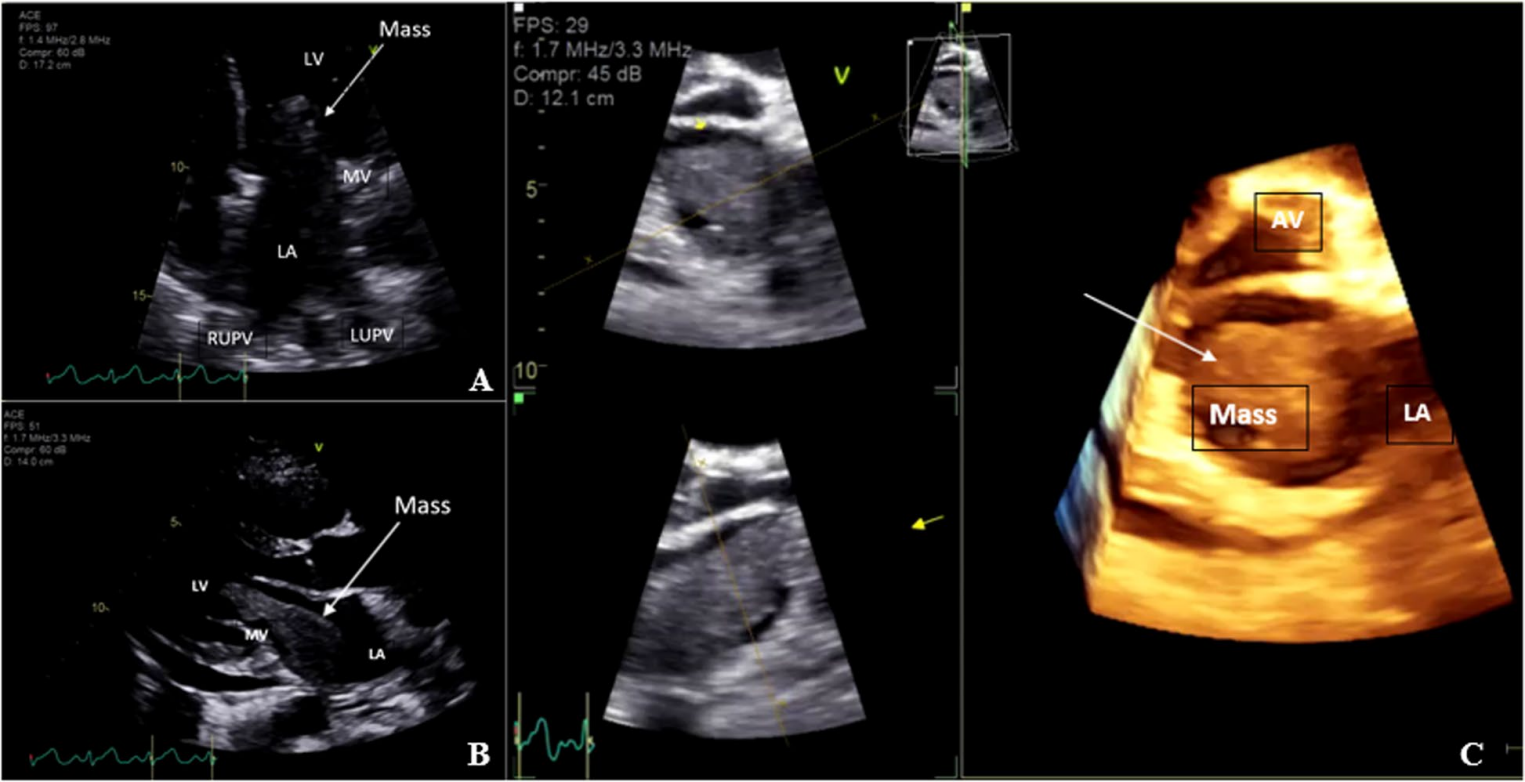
Transthoracic echocardiographic images of the left atrial mass. A 2D-echocardiography: Four chamber view demonstrates a large, echogenic mass attached to the lateral left atrial wall, prolapsing across the mitral valve causing left ventricular inflow obstruction. LA = left atrium, LV = left ventricle, MV = mitral valve, RUPV = right upper pulmonary vein, LUPV = left upper pulmonary vein. B 2D-echocardiography: Parasternal long axis view demonstrating obstruction of LV inflow with the mass prolapsing from the left atrium, across the mitral valve and into the left ventricle. C 3D-echocardiography demonstrating a large, well-circumscribed, echogenic mass (white arrow) filling the left atrium (LA). The aortic valve (AV) is oriented at the 12 o’clock position
